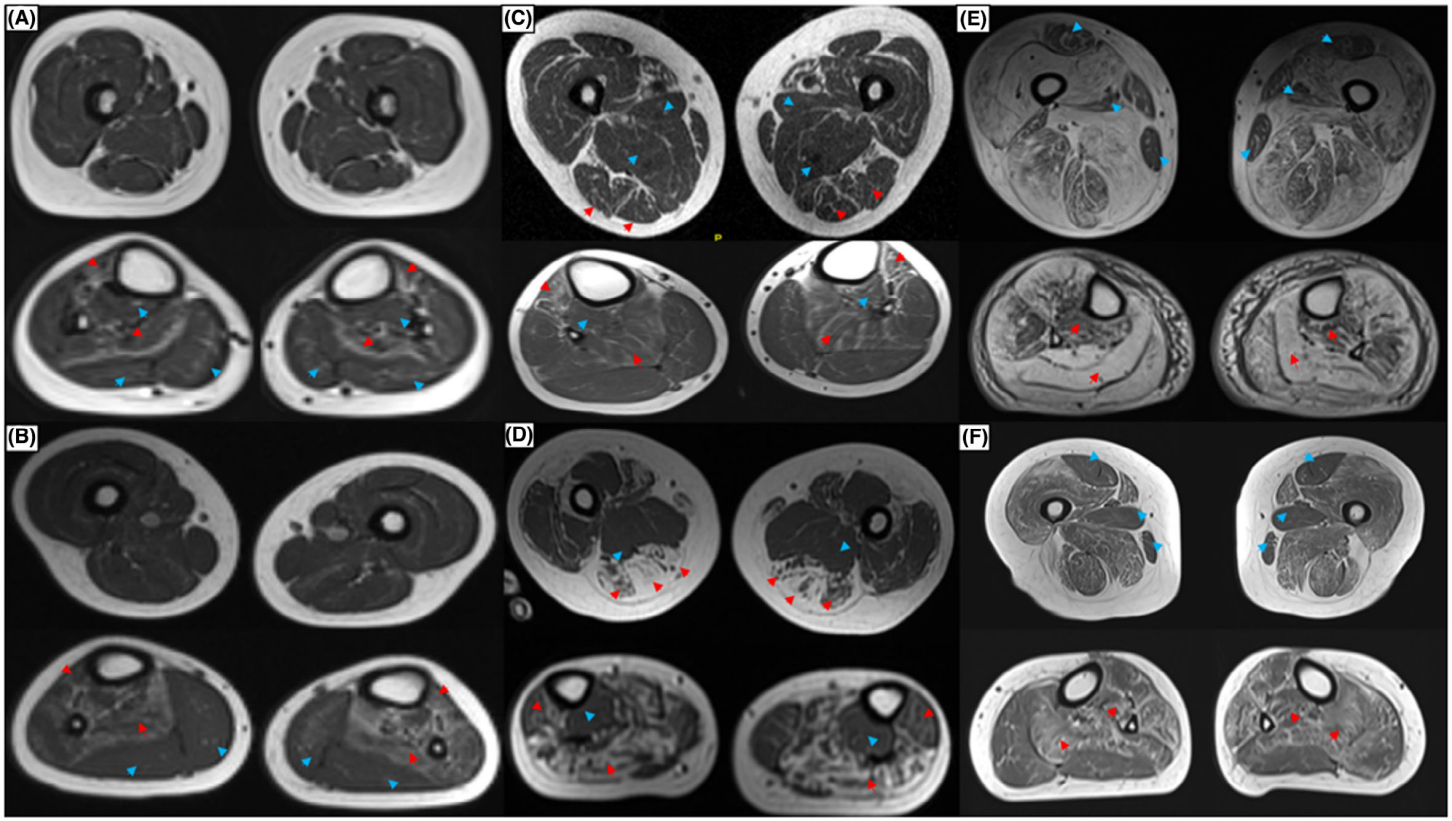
T1‐weighted lower limb MRI in NM patients from our cohort. Panels A (patient 2, 6 years old) and B (patient 1, 6 years old): *NEB*‐NM patients. Note the comparative sparing of the thigh muscles and the involvement of the tibialis anterior and soleus (red arrowheads) in both. Note the comparative sparing of the gastrocnemii in both and the tibialis posterior in patient 1 (blue arrow heads). Panels C (patient 11, 9 years old) and D (patient 15, 24 years old): *ACTA1*‐NM patients. Note the involvement of the hamstring muscles in the thigh (red arrow heads) and comparative sparing of the adductors (blue arrowheads). In the lower leg, note the involvement of the tibialis anterior and soleus (red arrowheads). Also, note comparative sparing of the tibialis posterior muscle (blue arrowheads). Panels E (patient 22, 60 years old) and F (patient 27, 51 years old): *TPM3*‐NM patients. Note the diffuse muscle involvement in the thigh with comparative sparing of the rectus femoris, gracilis and adductor longus muscles in both (blue arrowheads), and the involvement of the tibialis posterior and soleus muscles in the lower leg (red arrowheads).
